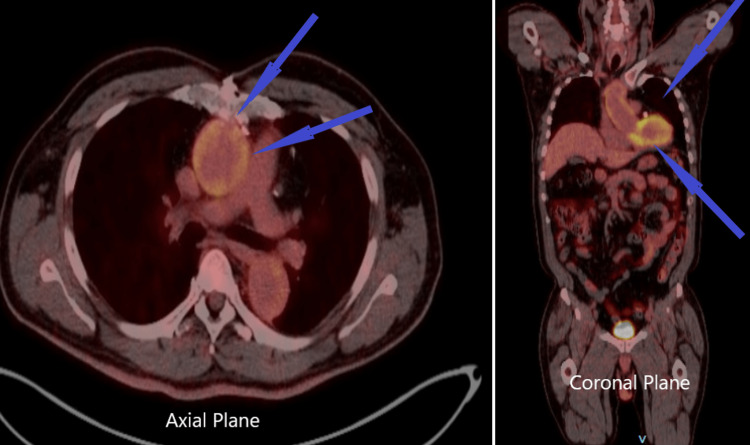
Axial planes and coronal planes of PET computed tomography, revealing moderately increased uptake of the aorta and its vessels PET: positron emission tomography
